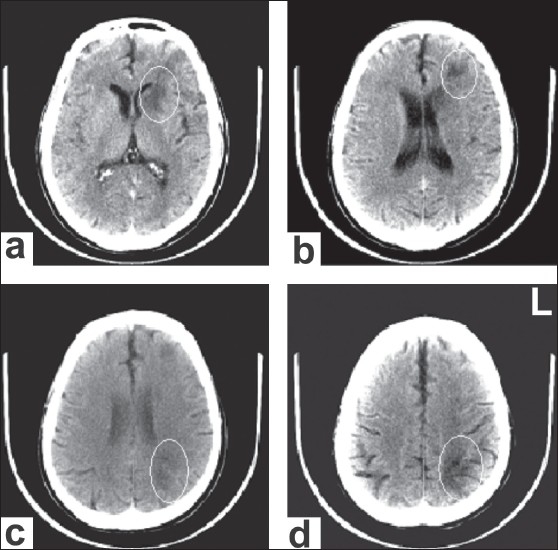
Plain axial CT showing hypodensity in the gray-white matter region of the left posterior parietal and frontal lobe, left caudate and lentiform nucleus, and the anterior limb of the internal capsule
